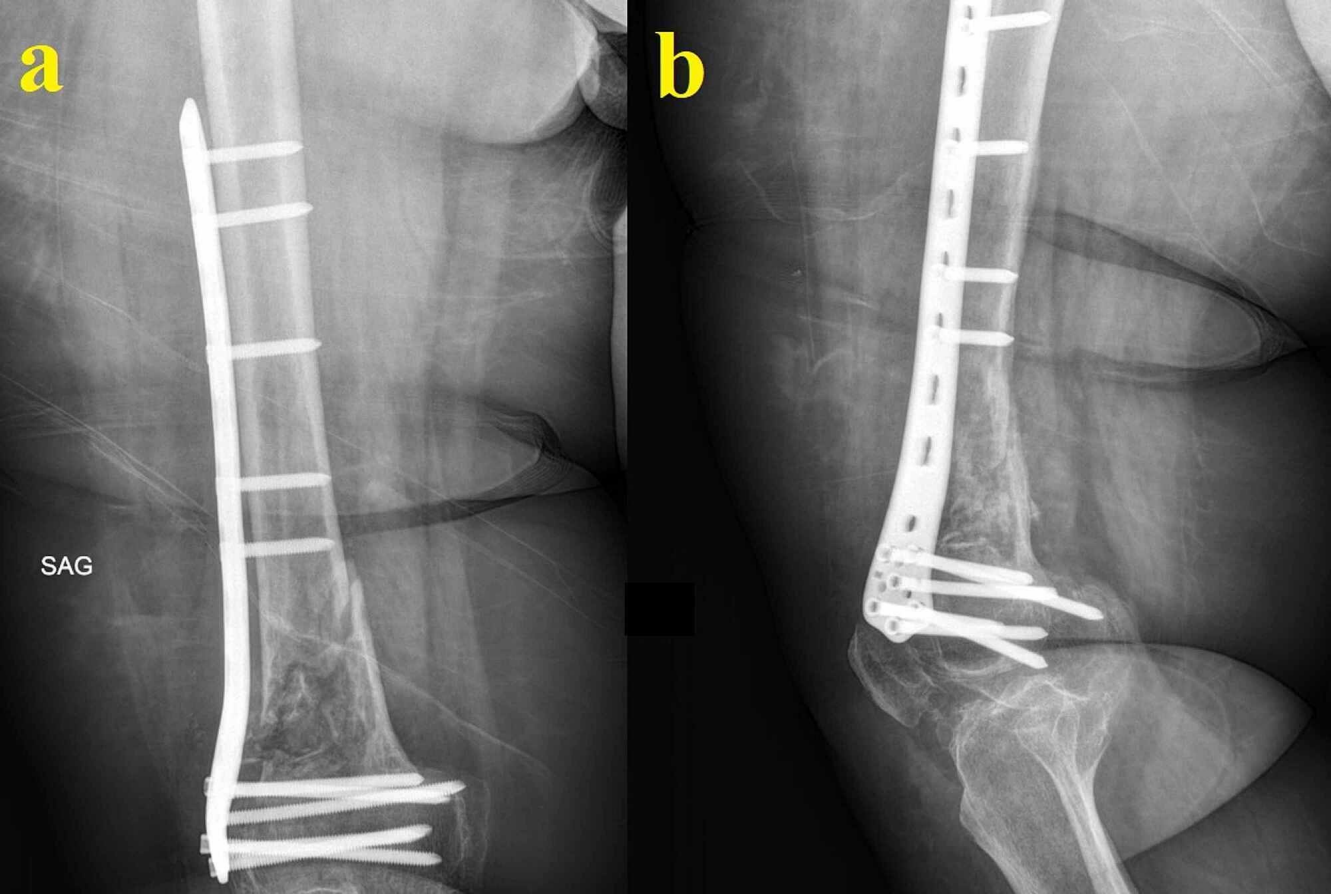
Radiographs in the second month postoperatively The patient has pain on ambulation. The radiographs show no signs of healing and reveal lysis and resorption around the fracture site
